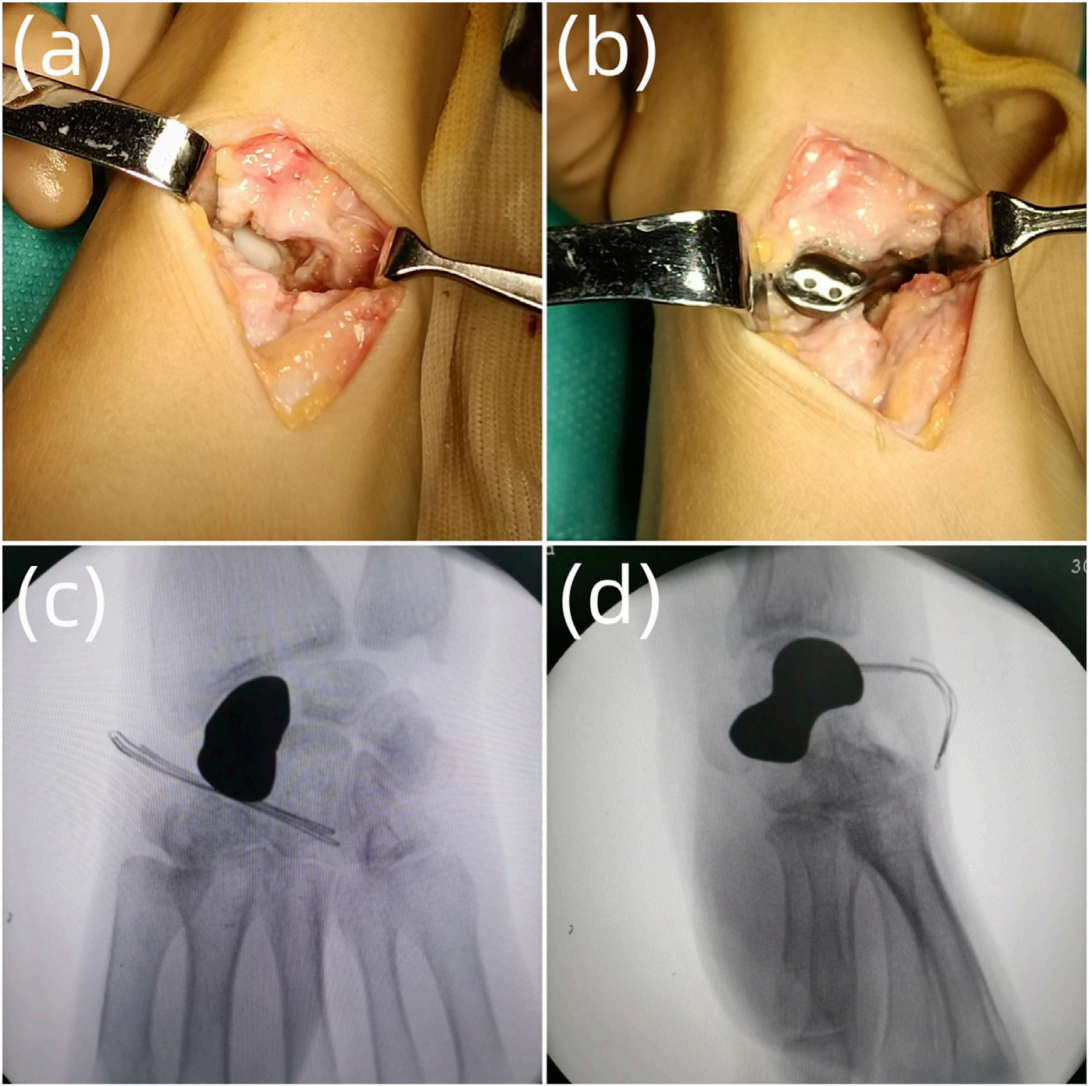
The surgical process. (A) Debridement of the scaphoid necrotic tissue; (B) Implantation of the scaphoid prosthesis; (C) Intraoperative anteroposterior radiograph of the scaphoid prosthesis after implantation; (D) Intraoperative lateral radiograph of the scaphoid prosthesis after implantation.
